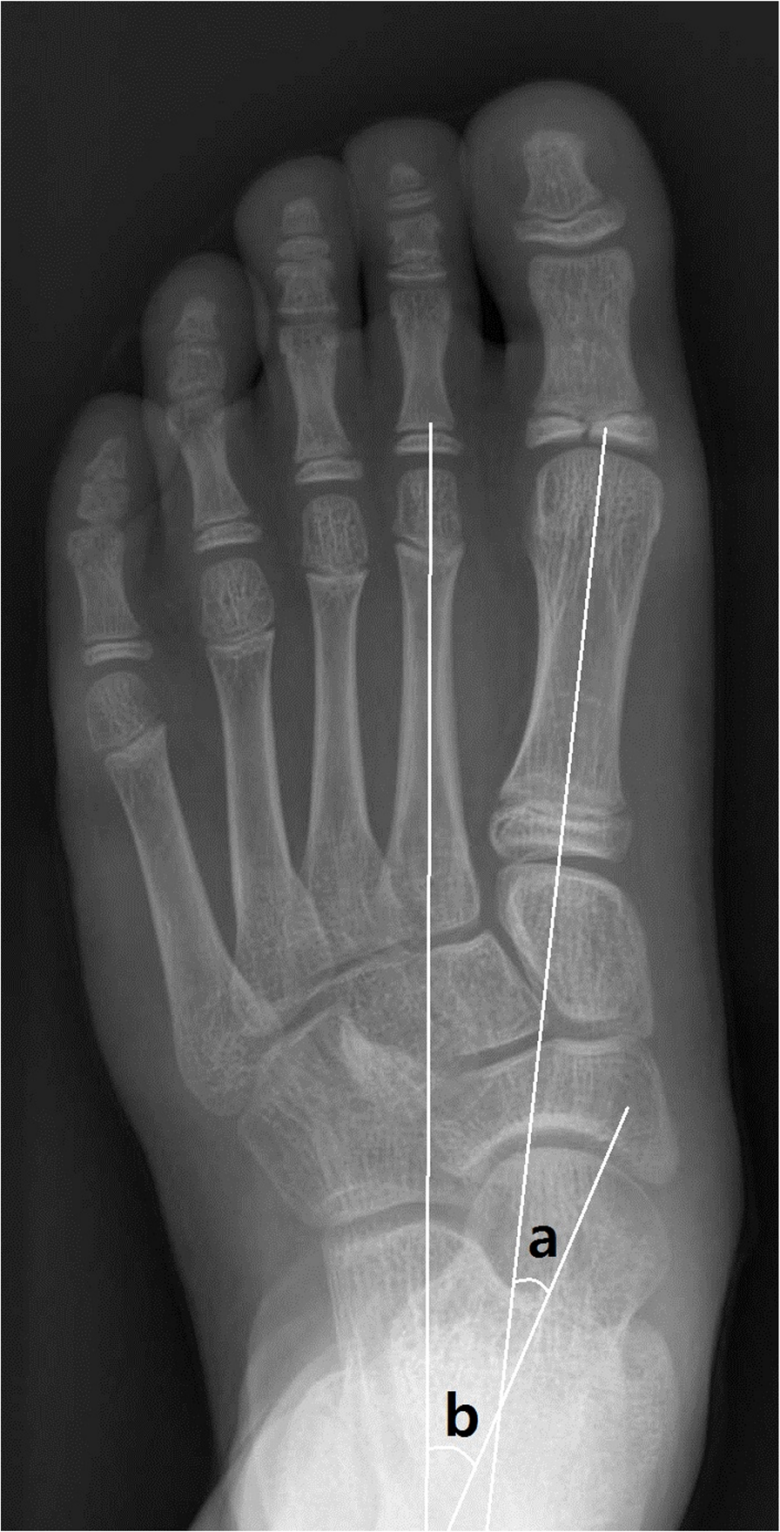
On weight-bearing anteroposterior (AP) foot radiographs, AP talo-1st metatarsal angle (a) and AP talo-2nd metatarsal angle (b) were measured
